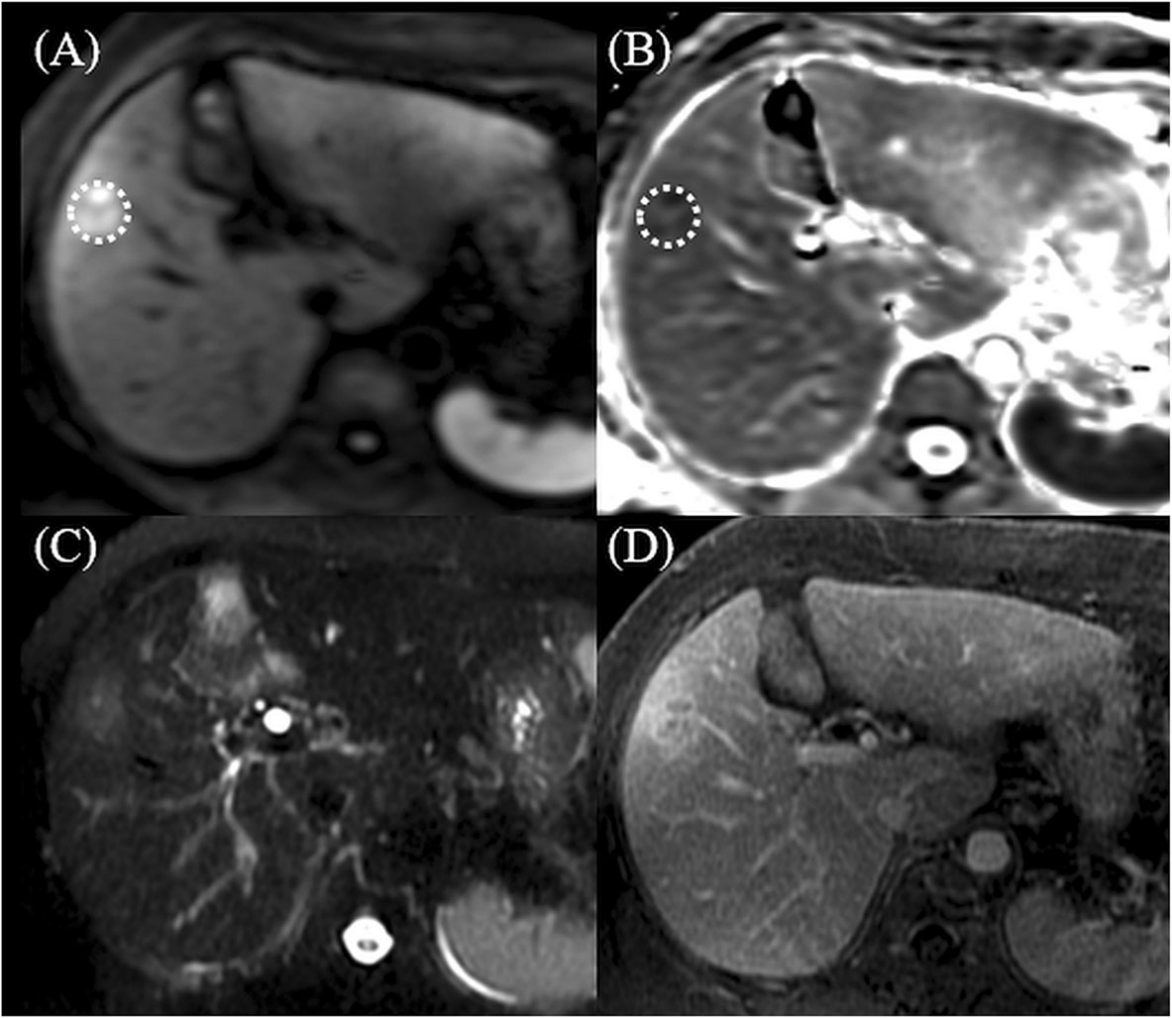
MR imaging in a 61-year-old woman. Pathologically confirmed grade 4 HCC. Dotted circles in a DWI and b ADC map show the placement of regions of interest. c T2-weighted imaging shows high signal intensity of the tumor. d Postcontrast T1-weighted image shows heterogeneous arterial enhancement with intralesional low SI area
